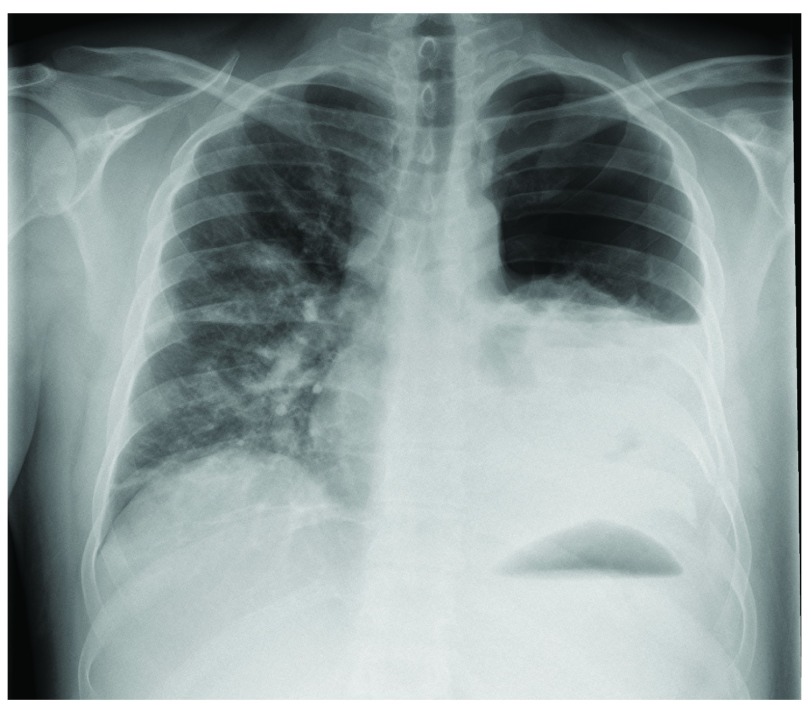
Chest radiograph showing a hemopneumothorax of the left lung.
